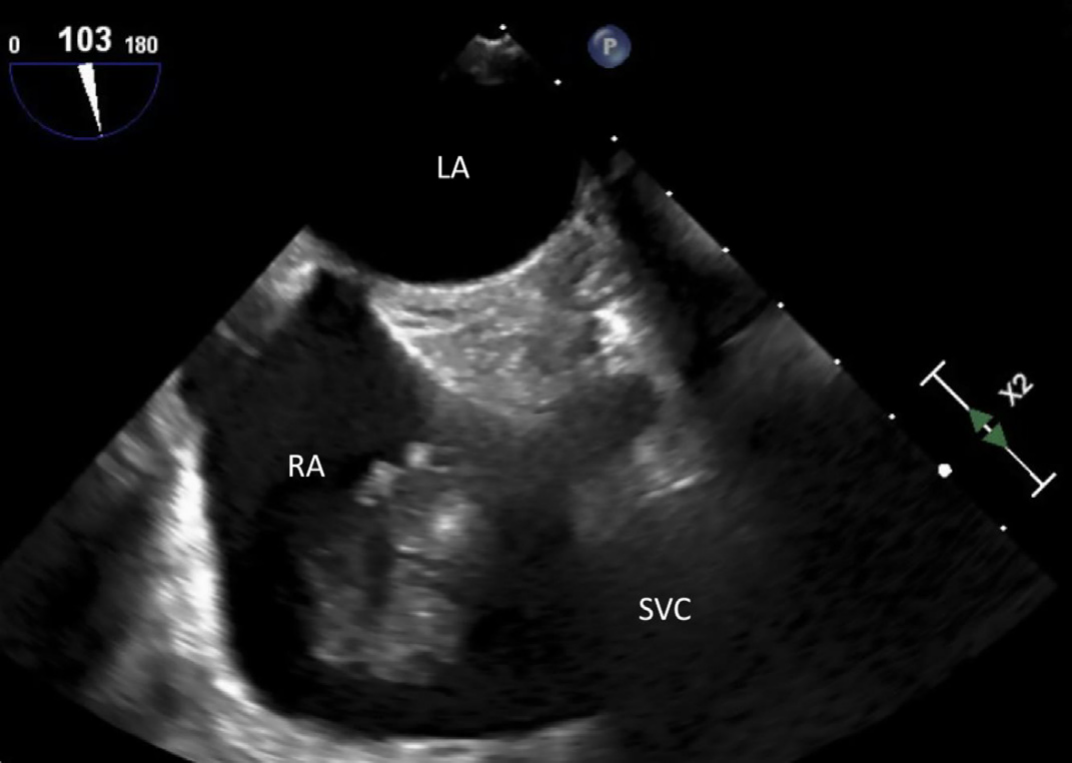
Transesophageal Echocardiography Showing a Right Atrial Mass LA = left atrium; RA = right atrium; SVC = superior vena cava.
